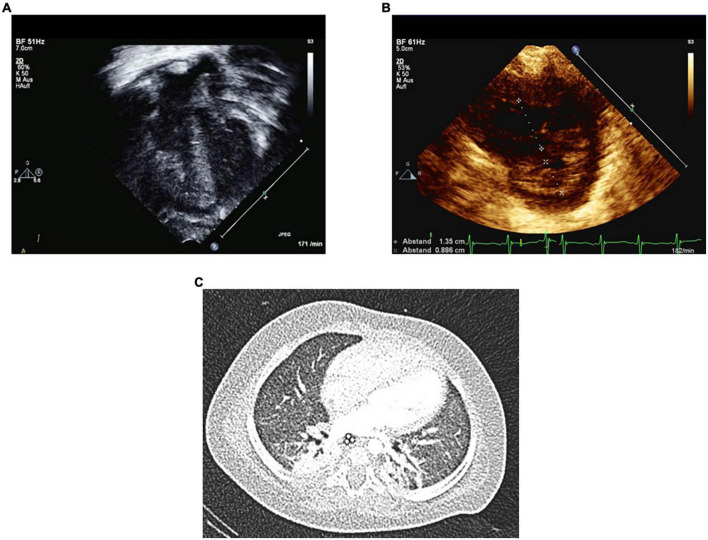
Echocardiography on day 2 and Chest CT on day 7 in PICU at the age of 6 months. Several Echo variables and ratios indicate severe PH on the second PICU day in acute cardiorespiratory decompensation. Panel (A) shows severe RV hypertrophy and RV dilation. Panel (B) shows increased RV/LV end-systolic diameter ratio of 1.5. Panel (C) shows chest CT with atelectasis of the right upper lobe and mild mosaic perfusion. No signs of cysts or bullae were identified.
